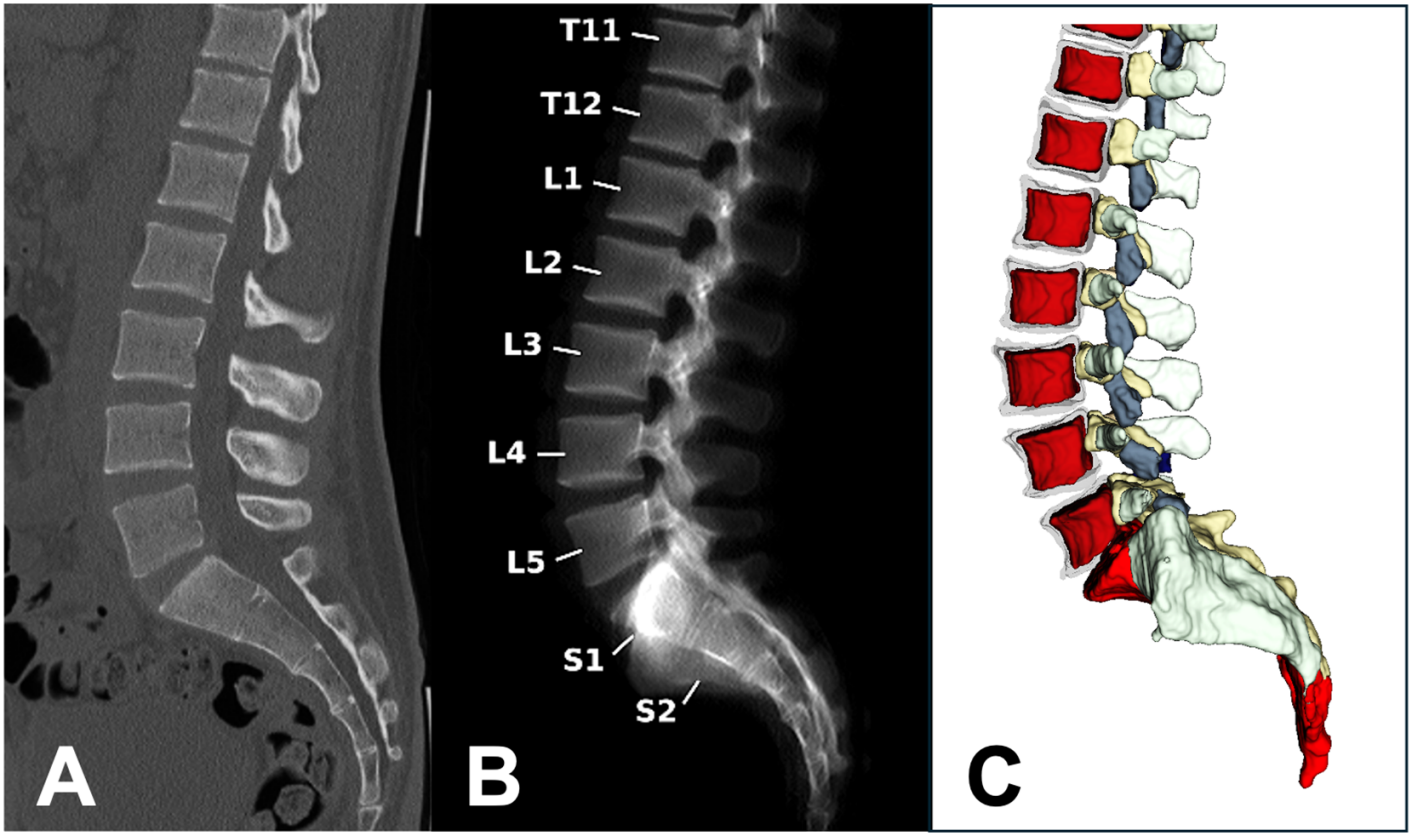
An exemplary case evaluated by SpineQ, showing a non-contrast enhanced CT scan of a 51-year-old female patient with a sagittal view of the thoracolumbar spine (A). Automated vertebral body labeling was performed (B) for vBMD measurements with asynchronous calibration. Spine segmentation, including subregion segmentation, was performed, and masks of the trabecular compartment (red) were obtained (C). Posterior elements and the sacrum were also routinely segmented (depicted in gray, yellow, and light red). For bone health evaluation, vBMD values of L1-3 were averaged, resulting in an average vBMD_L1-3_ = 104.9 mg/cm³ in this patient. CT, computed tomography; vBMD, volumetric bone mineral density.
